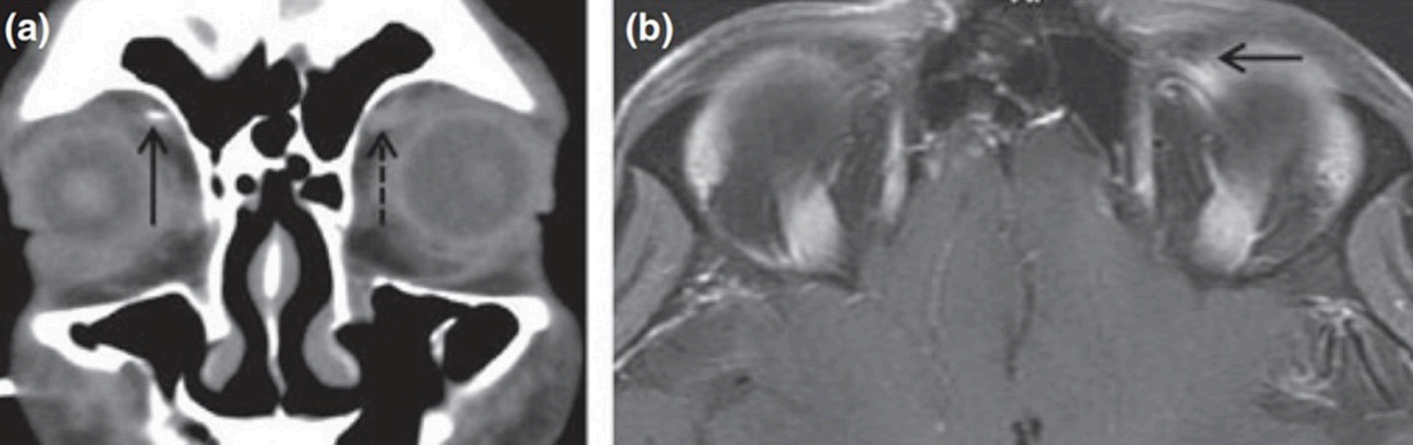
Computed tomography and magnetic resonance imaging findings of trochlear inflammation [adapted from Smith et al. (6) with permission granted by John Wiley and Sons]. (a) Coronal CT showing soft tissue enhancement of left trochlea (broken arrow) from acute inflammation. (b) MRI of orbits with gadolinium enhancement showing uptake at the left trochlea (solid arrow).
